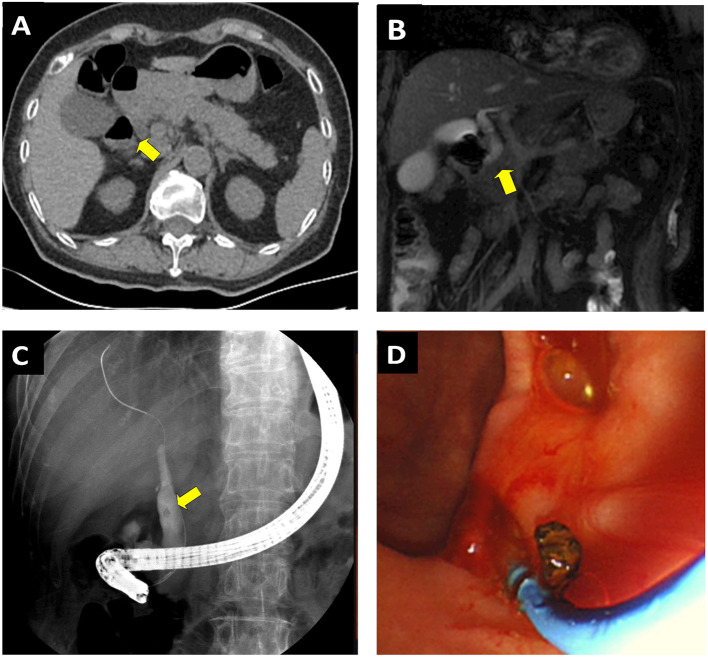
Performing ERCP on a 73-year-old female patient presented with acute pancreatitis secondary to JPDD related CBD stone. (A) CT scan shows the inflammation and swelling of the pancreas and a mildly enlarged gallbladder. A diverticular pouch was present at the junction of second and third portions of the duodenum (yellow arrows). (B) MRCP coronal haste thin slice image confirmed shows the presence of periampullary diverticulum, which causes extrinsic compression upon the CBD (yellow arrow). Note the possible CBD stone associated with dilation of the distal end of CBD. (C) ERCP confirm the CBD stone and divericulum exerting compression upon the CBD outlet. (D) The stone was successfully removed though the ERCP procedure.
